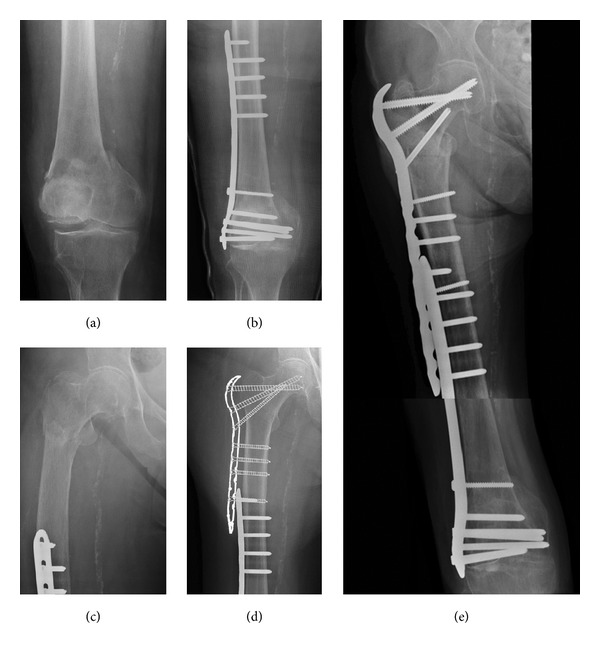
Case 3: (a) 1st fracture, (b) distal femoral plate (DFP) for supracondylar femoral fracture, (c) 2nd fracture-pertrochanteric, (d) using the prefracture X-ray for templating, (e) proximal femoral plate over DFP-healing of both fractures.
